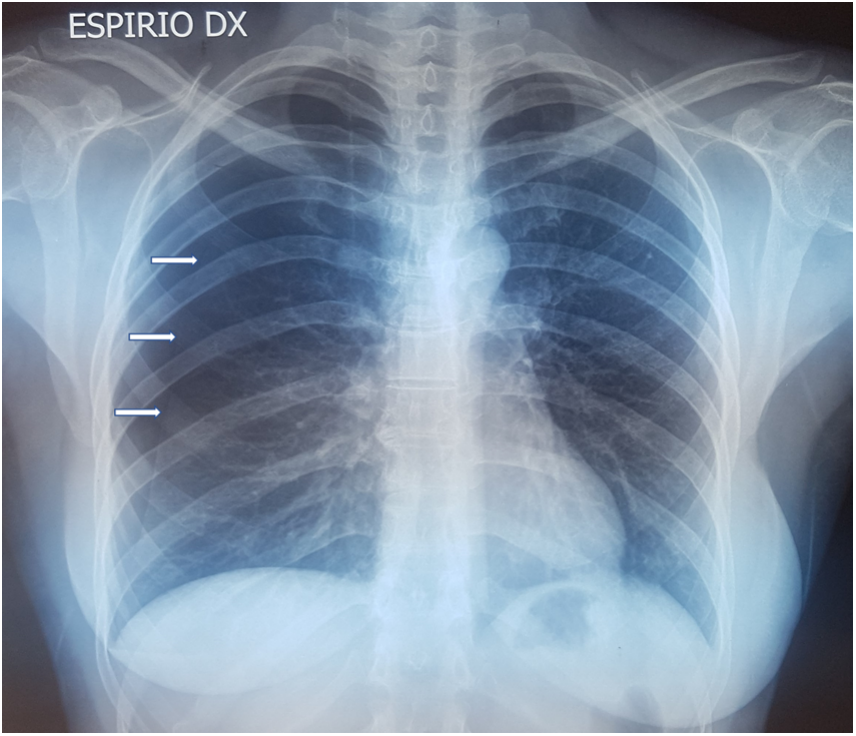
Chest x-ray showing CP on the right side: white arrows indicate the collapsed lung. CP, catamenial pneumothorax.
